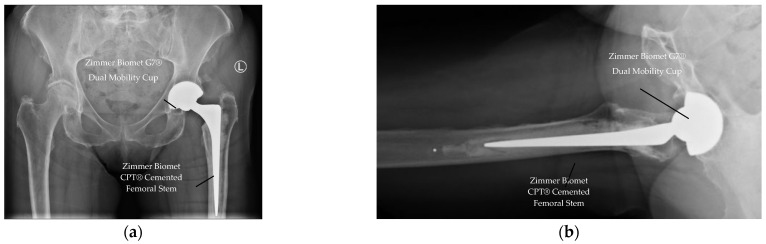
Postoperative radiographs at one-year follow-up. (a) Anteroposterior view showing well-positioned G7^®^ acetabular cup and cemented CPT femoral stem (left hip). (b) Lateral view confirming absence of radiolucent lines or implant migration.
